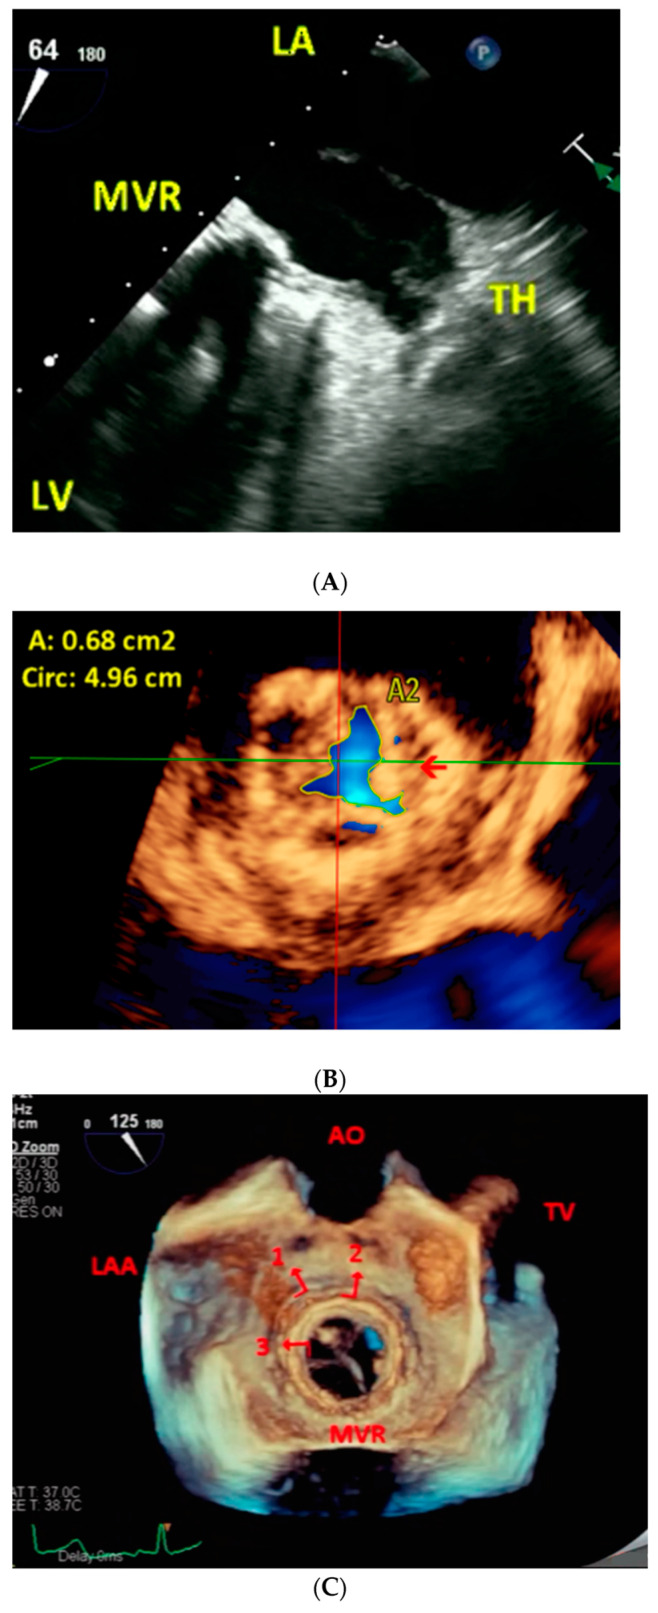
(A–C). The same patient (Case 2 above) returned 2 years and 8 months later with severe stenosis of the new MVR. Pre-procedure. Two-dimensional transesophageal echocardiography (A). A much smaller and poorly organized residual confined to LAA is noted in A. Live/real-time three-dimensional transesophageal echocardiography with color Doppler (B). The MVR orifice (red arrow) viewed in MPR mode in B measures 0.68 cm^2^ by direct planimetry indicative of severe restenosis. Post-procedure. Live/real-time three-dimensional transesophageal echocardiography (C). A short-axis view in full volume mode shows all the three MVR rings in C. # 1 points to the outermost ring of the original surgically implanted MVR. # 2 shows the middle ring of the first transcatheter implanted MVR. # 3 demonstrates the innermost ring of the second transcatheter implanted MVR. Abbreviations as in previous Figures.
